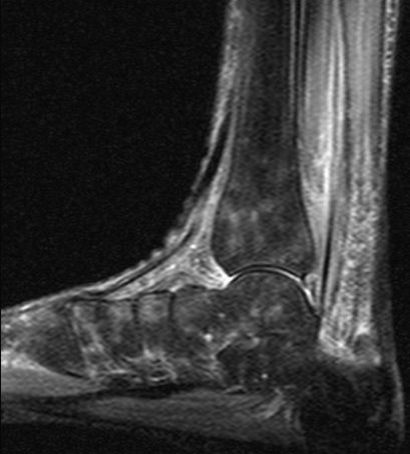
Same foot as in Figure 3. Follow-up MRI sagittal STIR-sequence shows patchy regredient bone marrow edema.
